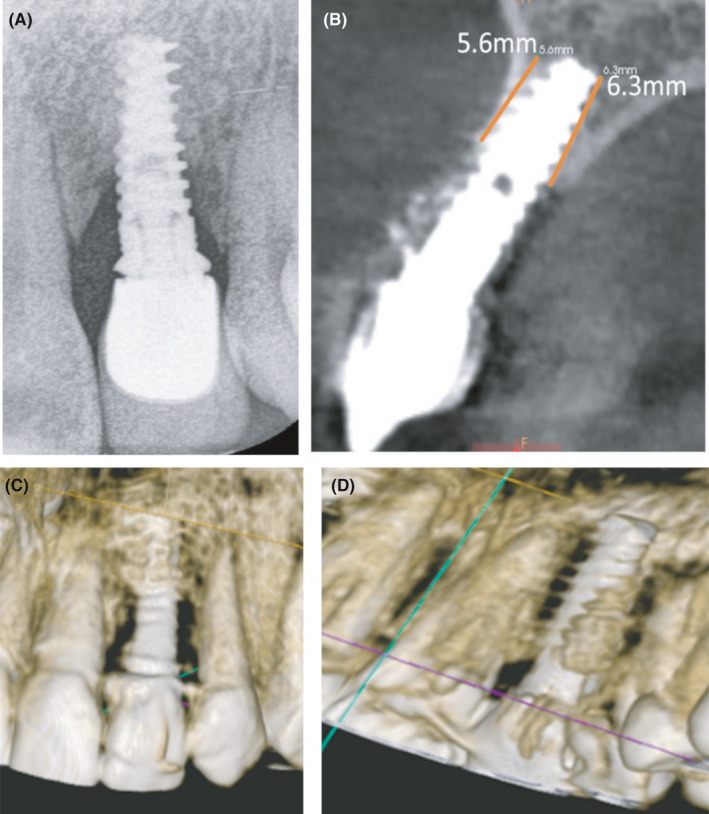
(A) Intraoral periapical radiograph of the implant site. (B) The CBCT imaging of implant site showing bone level in sagittal view. 3D composition of the implant site in buccal view (C) and palatal view (D)
